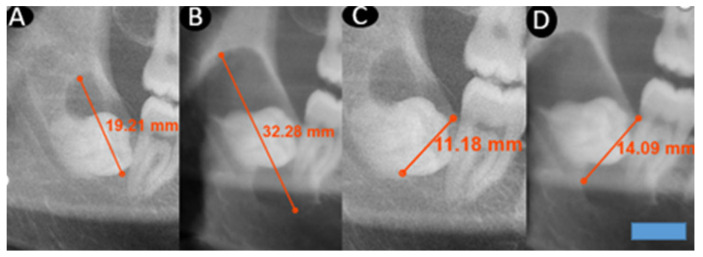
This figure demonstrates changes in cyst dimensions based on calibrated linear measurements obtained from panoramic radiographs at baseline and at long-term follow-up. The calibrated measurements highlight progressive enlargement over time and provide visual corroboration for the calculated growth rates derived from radiographic length assessments. A blue scale bar with a length of 10 mm and a height of 3 mm was added in the lower right corner of the image. (A): A–P first measurement. (B): A–P second measurement after 64 months. (C): I–S first measurement. (D): I–S second measurement after 64 months.
